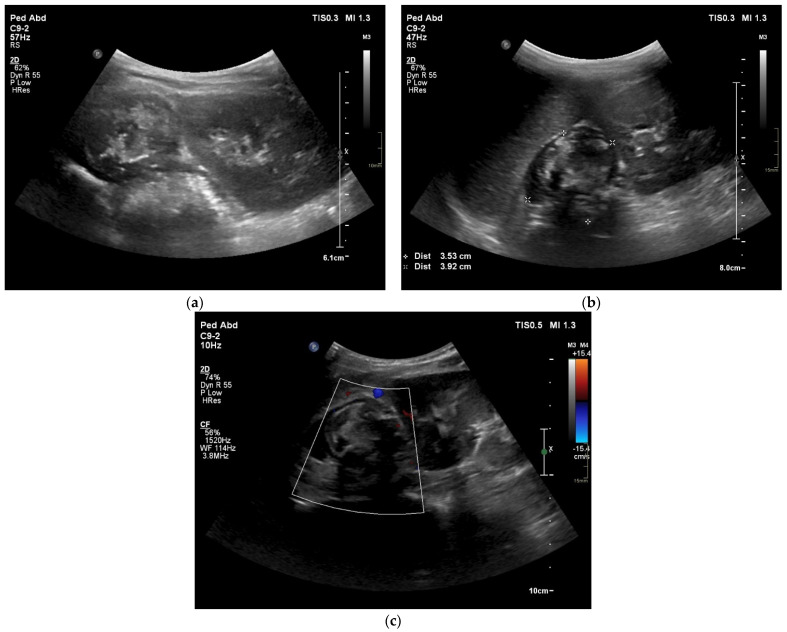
US examination showing (a) an echogenically heterogenous area with central hyperechoic reflections above the left kidney, (b) modeling of the left upper pole of the left kidney, (c) no visible vascular flow signals on Power Doppler.
